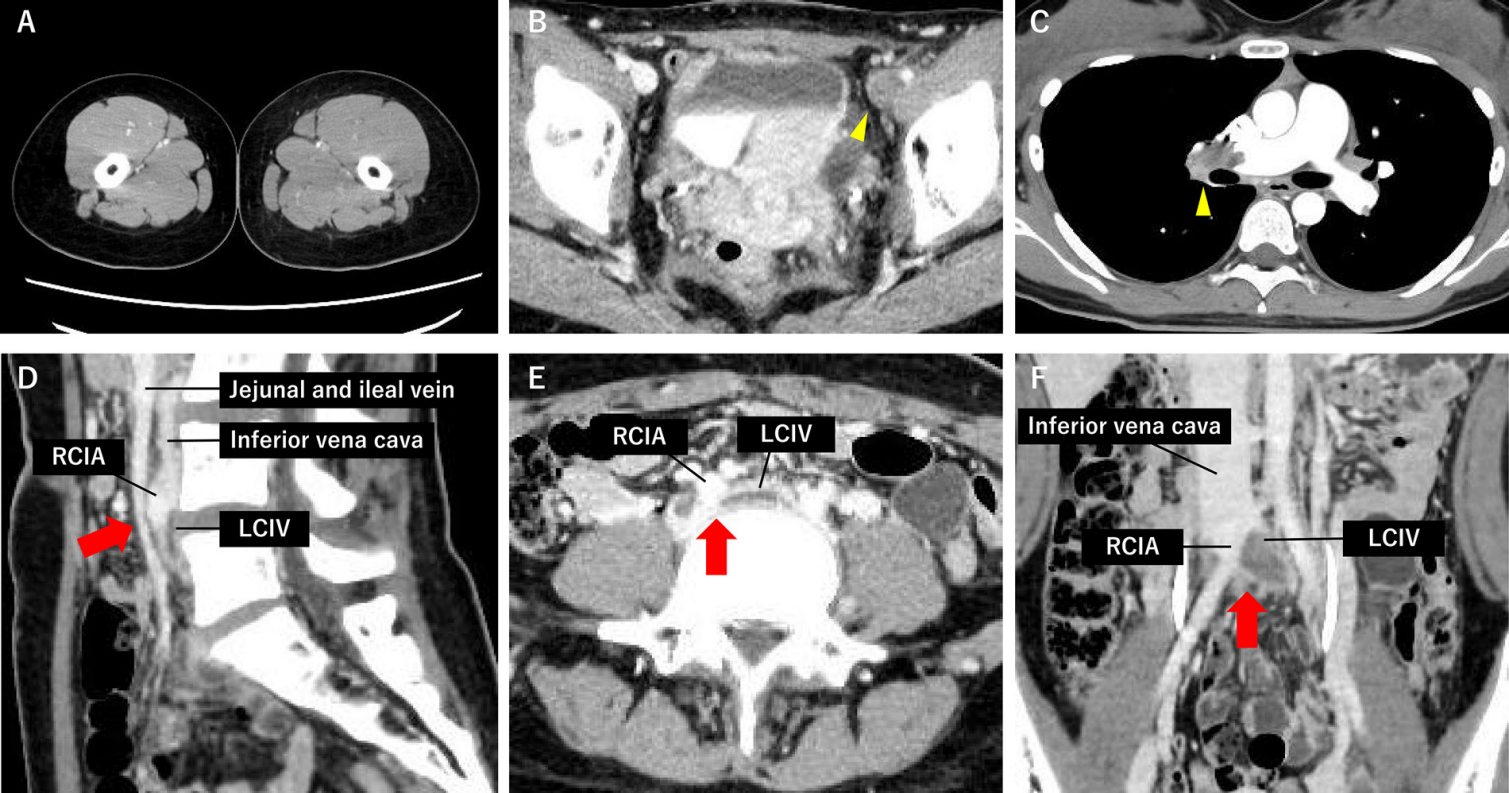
Initial CT angiography. (A) Left leg edema. (B) Left femoral venous thrombus (yellow arrowhead). (C) Right main pulmonary arterial thrombus (yellow arrowhead). (D and E) Left common iliac vein (LCIV) is compressed between the right common iliac artery (RCIA) and the fourth lumbar vertebra (red arrows). (F) Thrombi in the LCIV just distal to the compressive site by the crossing RCIA (red arrow).
